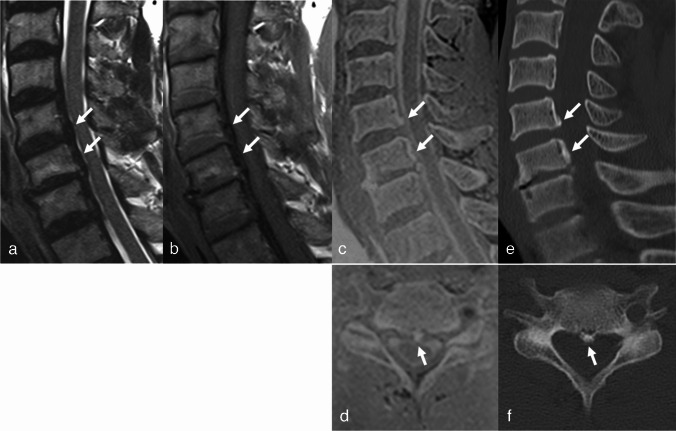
Fifty-eight-year-old male who presented with left upper extremity weakness. On sagittal T2- and T1-WI (a and b), a low-signal intensity band is seen between the posterior wall of the C5 and 6 vertebral bodies and dural sac (arrows). The sagittal 3D FLASH image (c) shows segmental OPLL at C5 and 6, which is confirmed on CT (e) (arrows). Note the OPLL at central zone of the C5 midbody on axial 3D FLASH (d) and CT images (f) (arrows). Both readers identified OPLL in the first and second reading, both increased their confidence grades from 1 to 3 after reviewing the additional 3D FLASH images in the second reading
